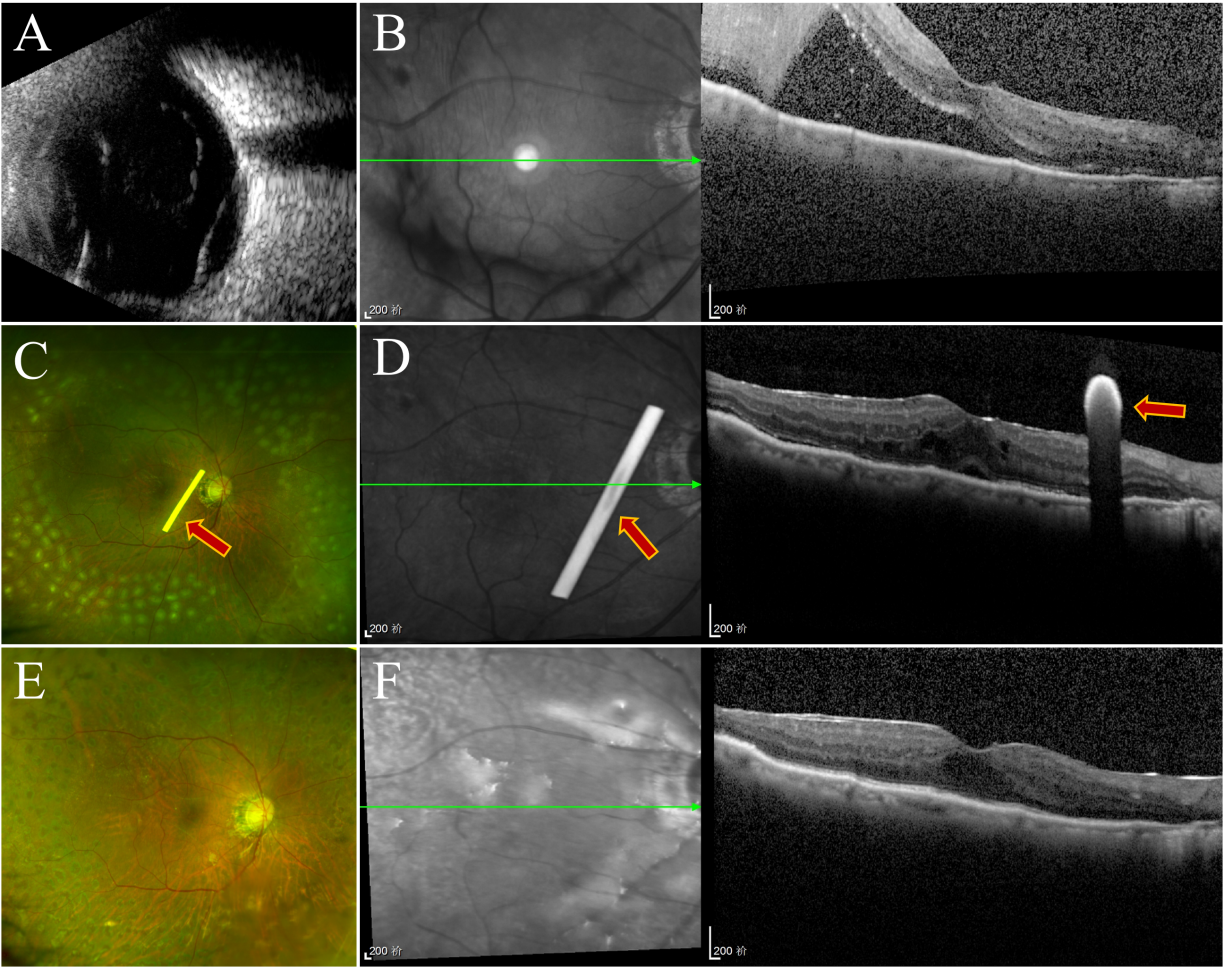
Follow-up examination of one patient in group 1. (A) Ultrasonography of the oculus dexter shows inferior retinal detachment. (B) Before surgery, OCT revealed retinal detachment. (C) SLO revealed silicone oil within the vitreous cavity, residual laser markings across the retina, and a white dexamethasone implant rod (marked with arrows). (D) OCT revealed that the retina was repositioned at the first week, and the dexamethasone implant was placed near the macular area (marked with arrows). (E, F) Three months later, the retina was repositioned, and the implant had been completely absorbed.
